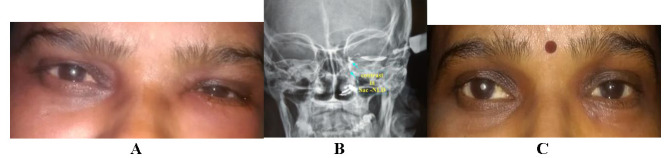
A-C A non-inflamed swelling over left lacrimal sac area; B. The swelling disappeared completely after four months after starting ATT; C. Contrast seen in NLD and inferior meatus on X-rays DCG
